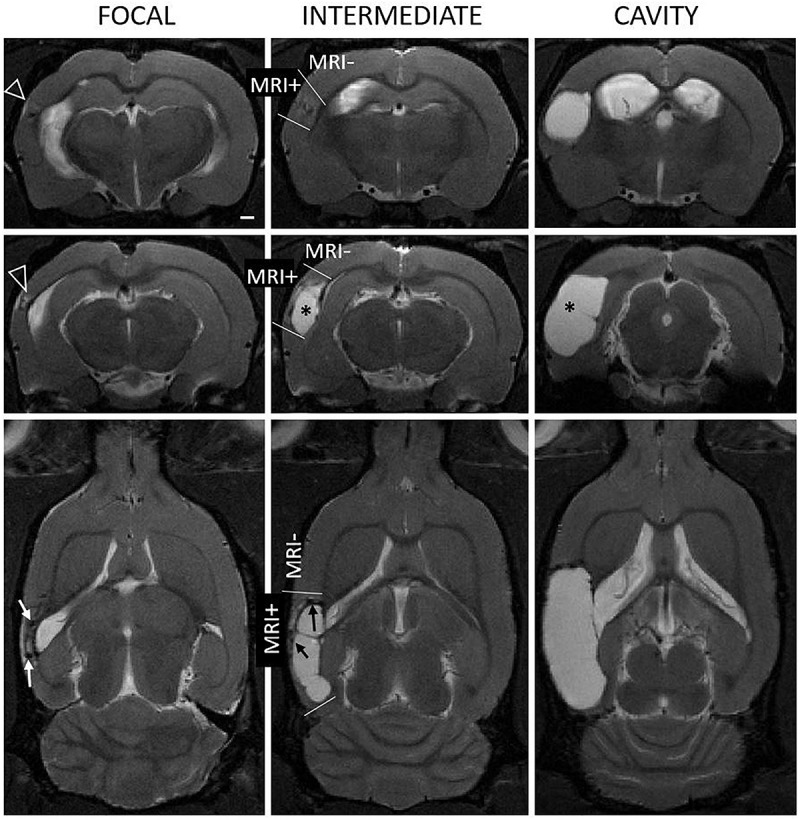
Heterogeneity of the endophenotype of cortical lesion in T2-weighted images at 1 month after lateral fluid-percussion induced TBI. Panel shows representative examples of “focal” (left column), “intermediate” (mid column), and “cavity-forming” (right column) endophenotypes, highlighting the 3D extent of the cortical lesions (see also Figure 3). Top row: Coronal views at the level of the rostral lesion tip. Note the rostral T2-enhancement (white triangle). Middle row: Center of the cortical lesion. Bottom row: Horizontal view depicting the rostro-caudal lesion extent. Contusion site is atrophied and the cavity filled with cerebrospinal fluid (asterisk). In focal and intermediate cases, the lesion was still expanding at 1 month and showed prominent T2w hyperintensity in the surrounding cortex, suggesting on-going inflammation (open arrowheads). We also found hyperintensity in association with hypointense hematomas or iron deposits (black and white arrows). MRI negative (MRI–) cortex refers to areas with normal gray matter contrast while MRI positive (MRI+) cortex refers to all observable abnormalities. Scale bar equals 1 mm.
